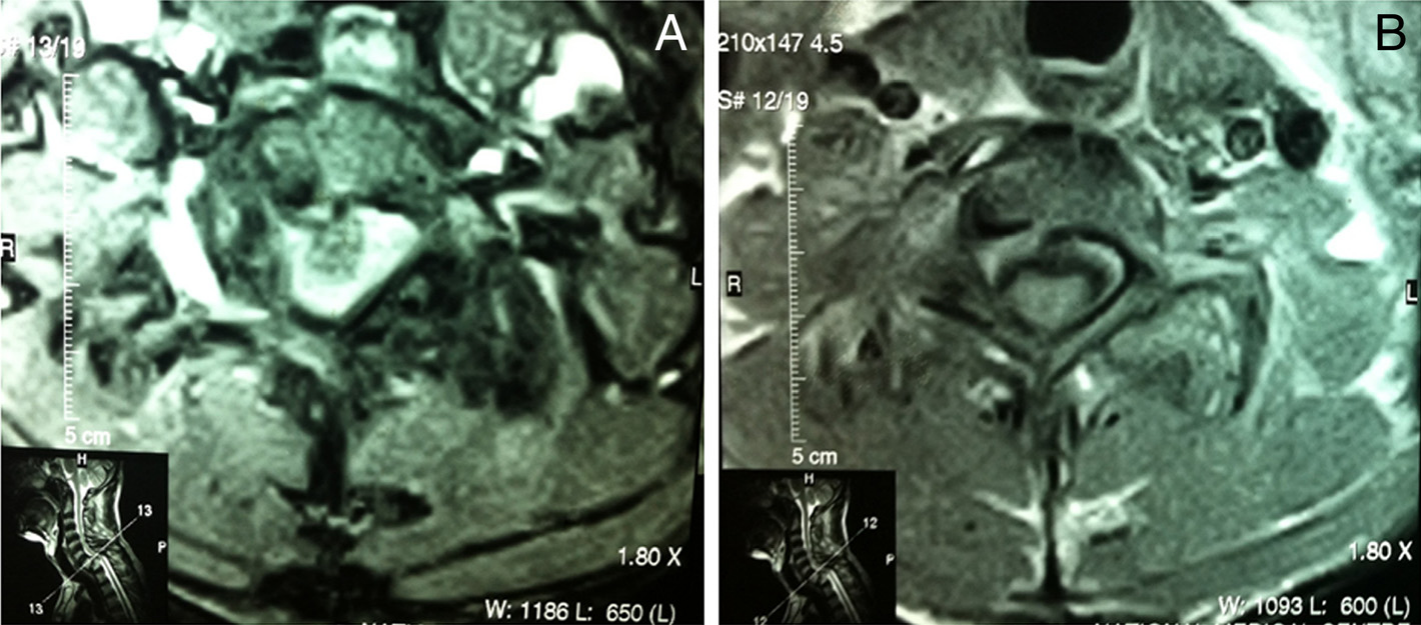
Computed tomography cervical spine axial images. Computed tomography images with (A) and without (B) contrast reveal subluxation of C7 and a major compression of the spinal cord.
